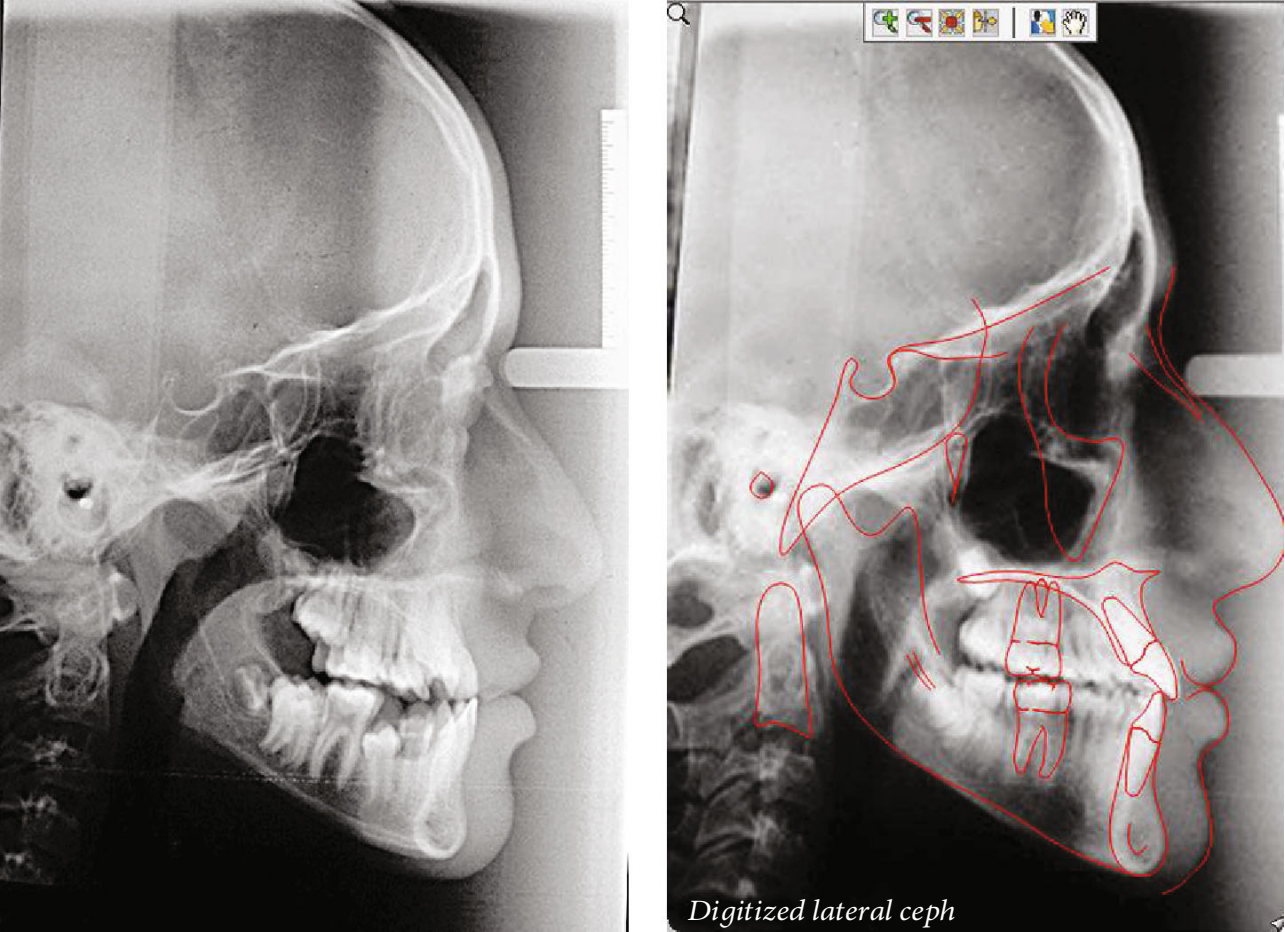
Posttreatment cephalometric radiograph and its tracing.
